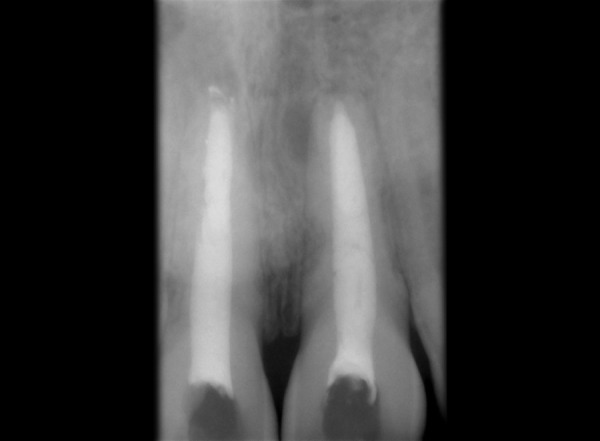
Radiographic appearance at 12 months
